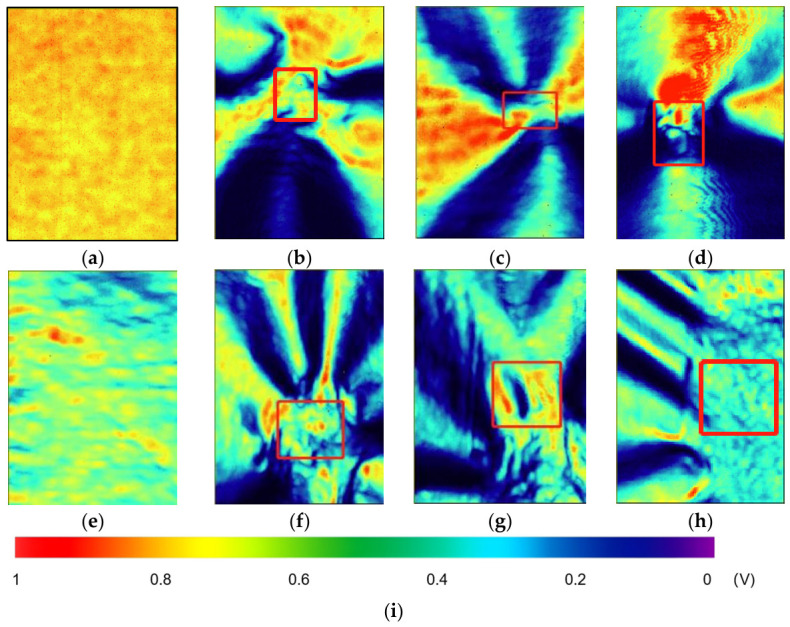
C-scan imaging results of roughening degree of different paper materials, The red box in the figure indicates the naturally dried area after water dripping, which is the region where the coarsening phenomenon occurs: (a) newsprint with 0.22 g/m^3^ water content; (b) newsprint with 625 g/m^3^ water content; (c) newsprint with 1250 g/m^3^ water content; (d) newsprint with 2500 g/m^3^ water content; (e) Xuan paper with 0.22 g/m^3^ water content; (f) Xuan paper with 625 g/m^3^ water content; (g) Xuan paper with 1250 g/m^3^ water content; (h) Xuan paper with 2500 g/m^3^ water content. (i) pseudo-color-Color bar corresponding to ultrasound amplitude values.
